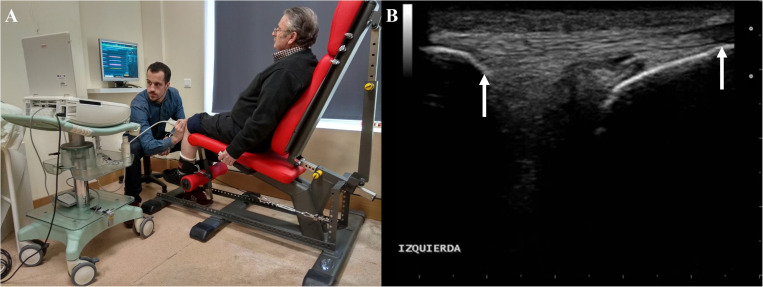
Demonstrative example of the experimental setting for acquisition of *in vivo* patellar tendon behavior during isometric ramp contractions (A). Field of view of ultrasound images collected from the patellar tendon (B). White arrows indicate points on patella (left) and tibial tuberosity (right) that will be tracked to determine tendon elongation.
